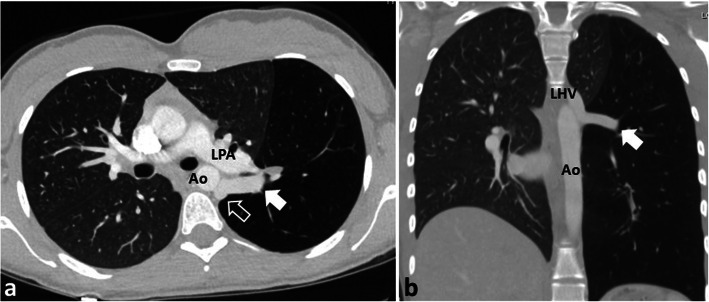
Chest CT scan, (a) axial view, the white arrow pointing to the inferior pulmonary vein draining into the left hemi-azygos venous system (outlined arrow), and (b) coronal view confirming the venous drainage into the systemic circulation. (Ao = descending aorta; LHV = left hemi-azygous vein; LPA = left pulmonary artery)
